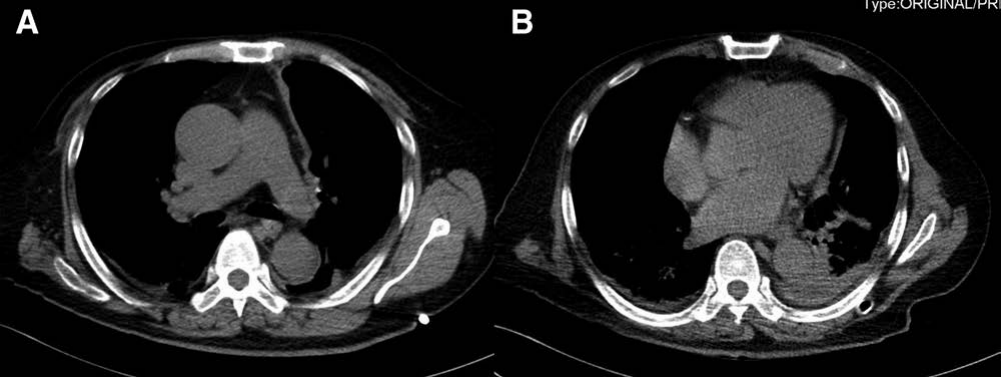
Image of Thoracic CT. (A and B) Thoracic CT scan on d 18 of hospitalization. The image showed that the left pleural effusion was obviously absorbed. CT = computed tomography.
